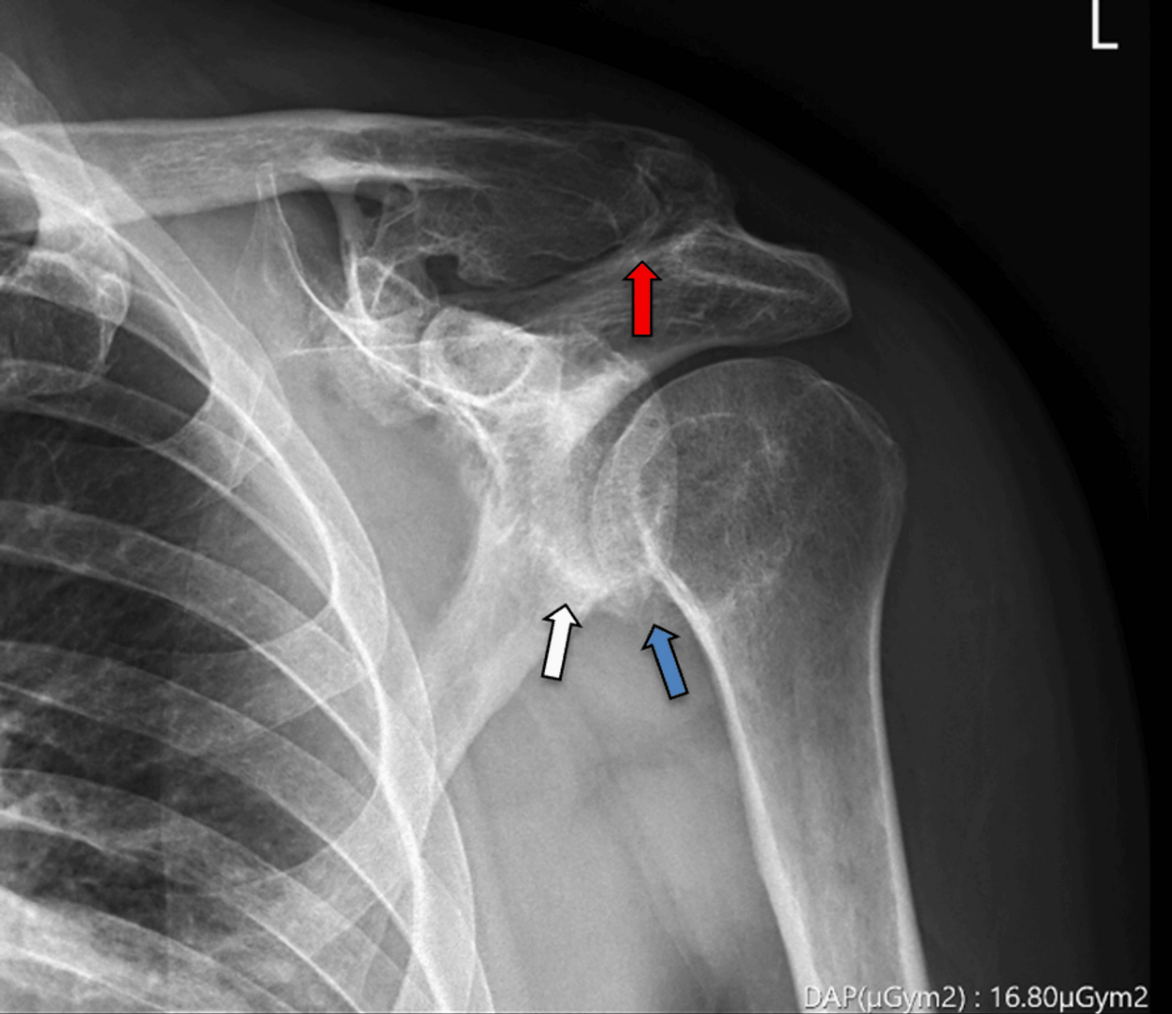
Standard anteroposterior shoulder radiograph Acromioclavicular (red arrow) and glenohumeral osteoarthritis findings with prominent osteophyte formation (blue arrow) and subchondral sclerosis (white arrow).
